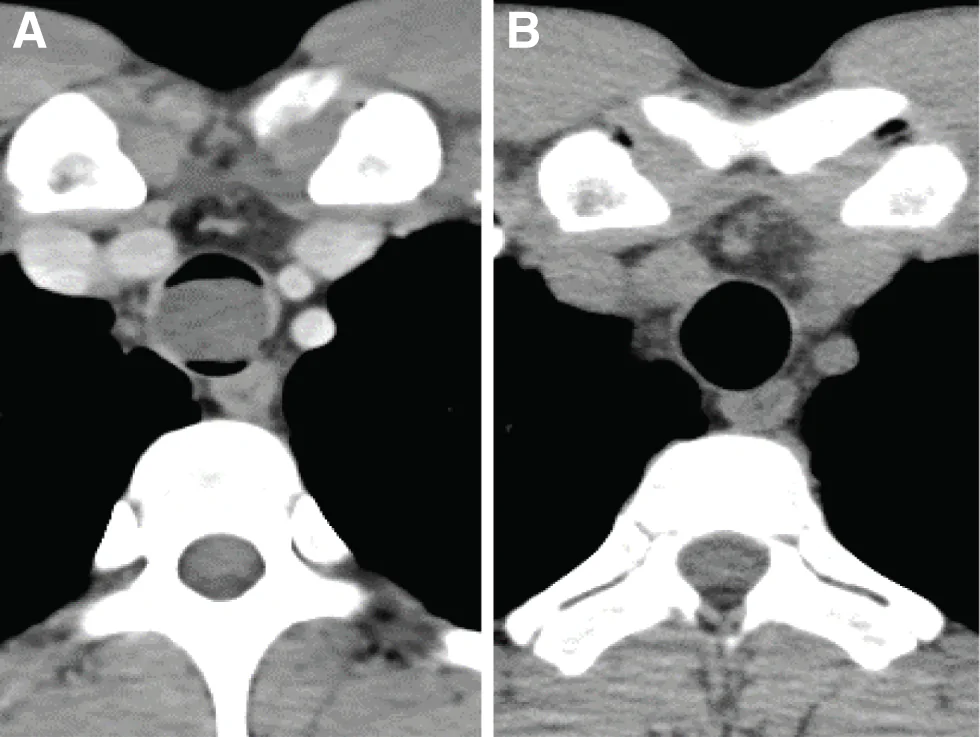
CT findings. (A) Preoperative chest CT. A 2.2 × 2.0-cm intraluminal tumor is observed in the trachea approximately 6.0 cm above the carina. (B) Postoperative chest CT. No definite residual tracheal lesion is observed after bronchoscopic resection.
